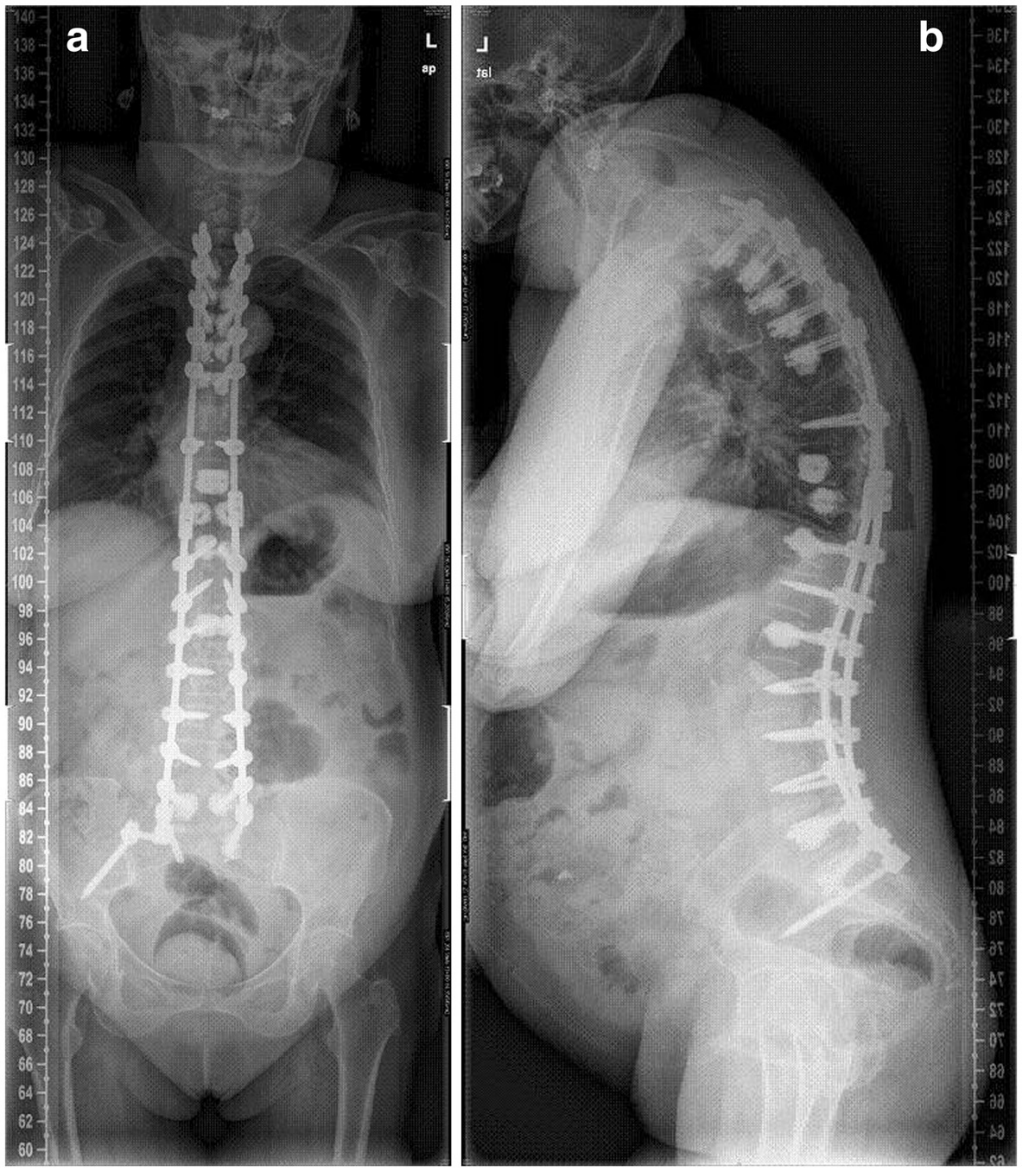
Post-revision surgery p-a (a) and lateral (b) x-ray. The fusion was extended to T2 with decompression at T9-T10, using bilateral pedicular screws augmented with cement at T2, T3, T4, T5 and T6. Satisfactory profile in both frontal and sagittal planes were obtained.
